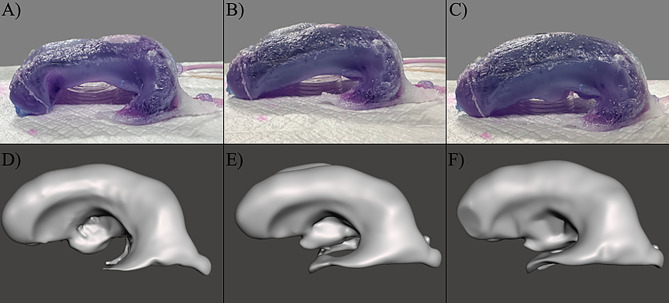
Qualitative profile comparison of the *P-5* ventricle model to its clinical counterpart. (A) The *P-5 (II)* manufactured ventricle model filled with approximately 198 mL of fluid (B) The ventricle model filled with approximately 275 mL of fluid (C) The ventricle model filled with approximately 321 mL of fluid (D) The *P-5 (III)* 3D ventricle model derived from a third consecutive MR image with a calculated internal volume of 198 mL (E) The *P-5 (II)* 3D ventricle model derived from a second consecutive MR image with a calculated internal volume of 275 mL (F) The *P-5 (I)* 3D ventricle model derived from a first of three consecutive MR image with a calculated internal volume of 321 mL. The volumes of fluid in each ventricle model corresponded to the volumes of the patient’s lateral and third ventricles at the time of scanning, calculated from MR images
